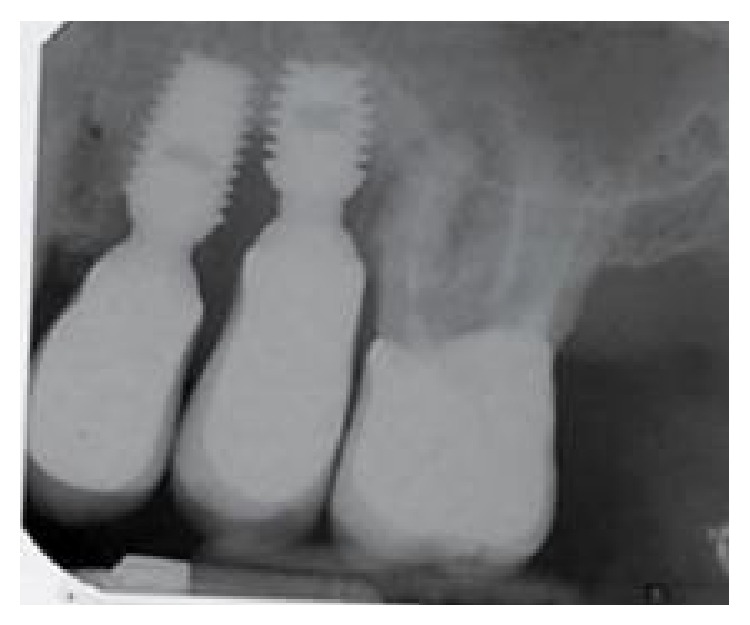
The baseline periapical radiograph indicated a deep interproximal peri-implant bone lesion.
